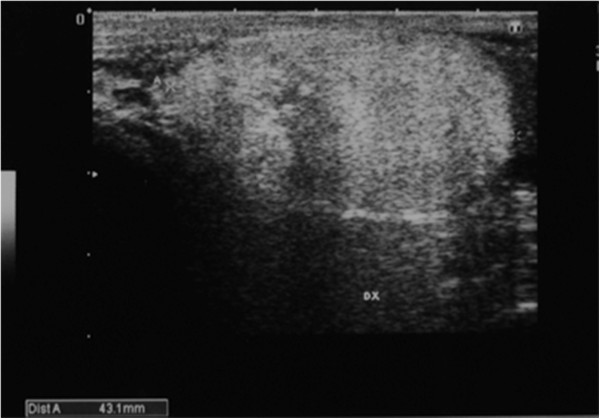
Ultrasound scan showing a 4.3 × 2.3 cm non
